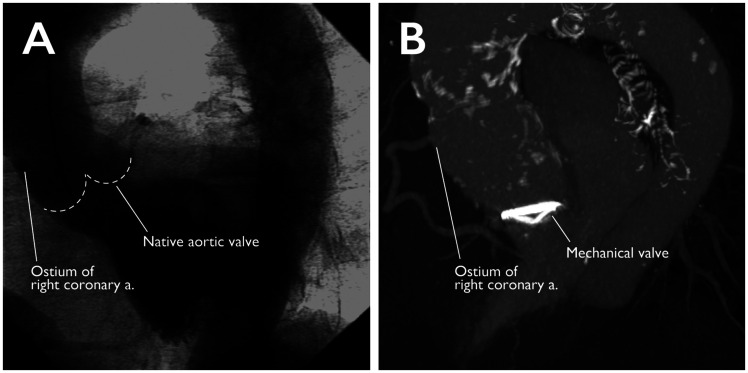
Morphological changes in the sinus of Valsalva. (A) The left ventriculogram before aortic valve replacement at age 59. The sinus of Valsalva, whose morphology is close to normal, can be observed. (B). A preoperative four-dimensional computed tomography at 74 years of age showing vertical dilation of the sinus of Valsalva; the height of the right coronary artery ostium remains changed from A and B (Th 8), indicating that the Valsalva's sinus is dilated in the left ventricular direction.
